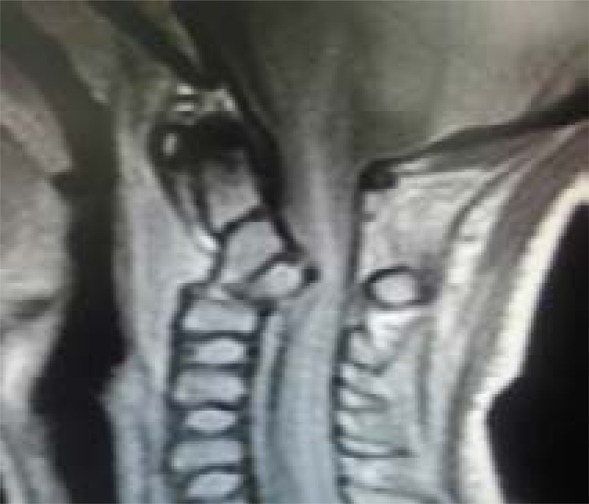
Sagittal cervical proton density weighted (PDW) MRI on day 3 post trauma/admission showing an intact but displaced C2-C3 disc protruding into the intraspinal canal and causing cord compression.
